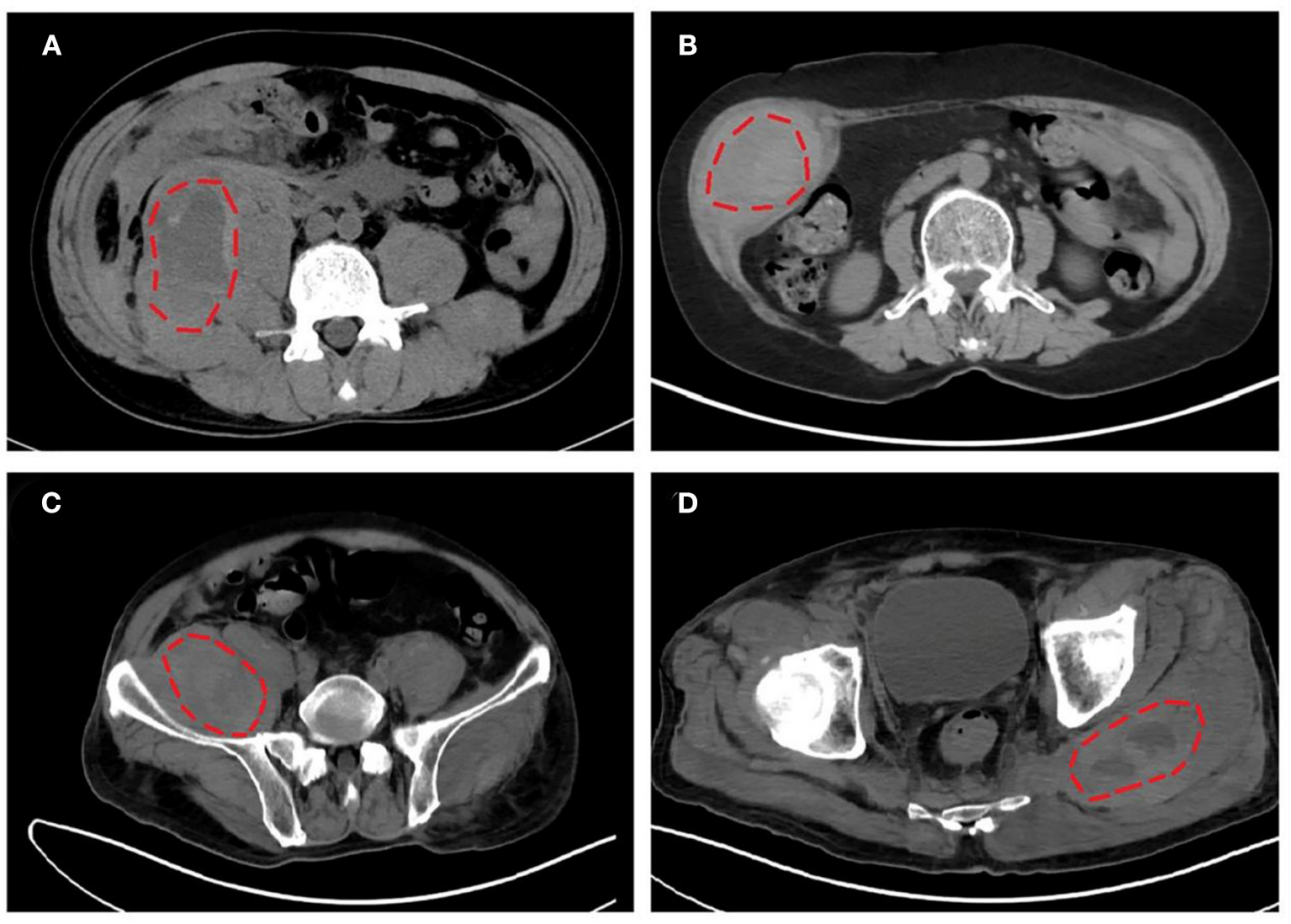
Representative computed tomography (CT) images of intramuscular hematomas in our cases. (A) Hematoma in right iliopsoas and psoas of listed case no. 17. (B) Hematoma in right musculi obliquus internus abdominis of listed case no. 18. (C,D) Hematomas in right iliopsoas and left gluteus maximus of listed case no. 20.
